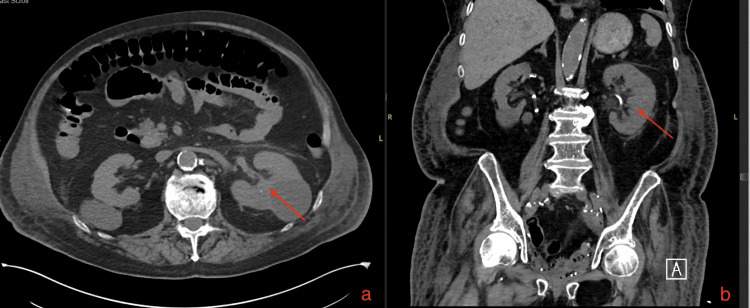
A CT scan of his abdomen pelvis shows clearance of gas and normal left renal parenchyma demarcated by a red arrow. (a) A CT scan of the axial section showing clearance of gas. (b) A CT scan of the coronal section showing clearance of gas.
